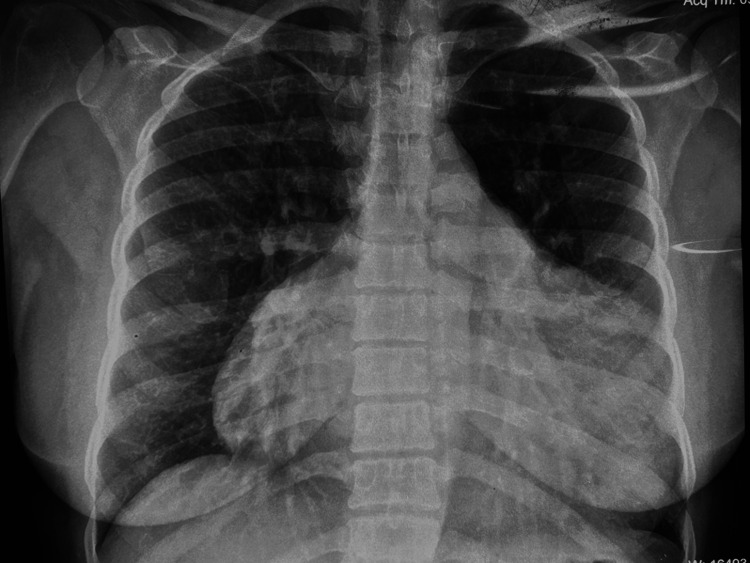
Chest radiograph (anteroposterior view) showing an enlarged cardiac silhouette (“water-bottle” sign of pericardial effusion).
